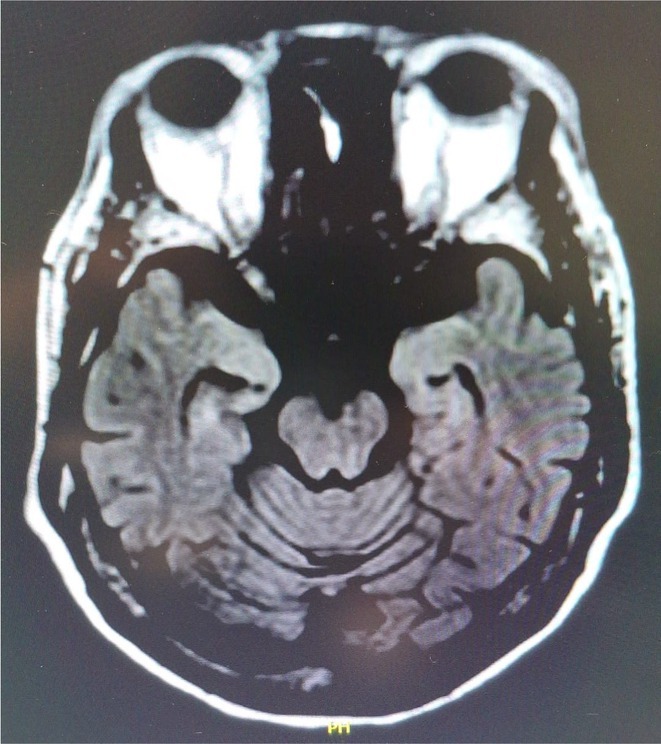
FIGURE 2 Brain MRI of the second patient showed bilateral hippocampus hyperintensity associated with mild volume loss, compatible with subacute autoimmune encephalitis.
